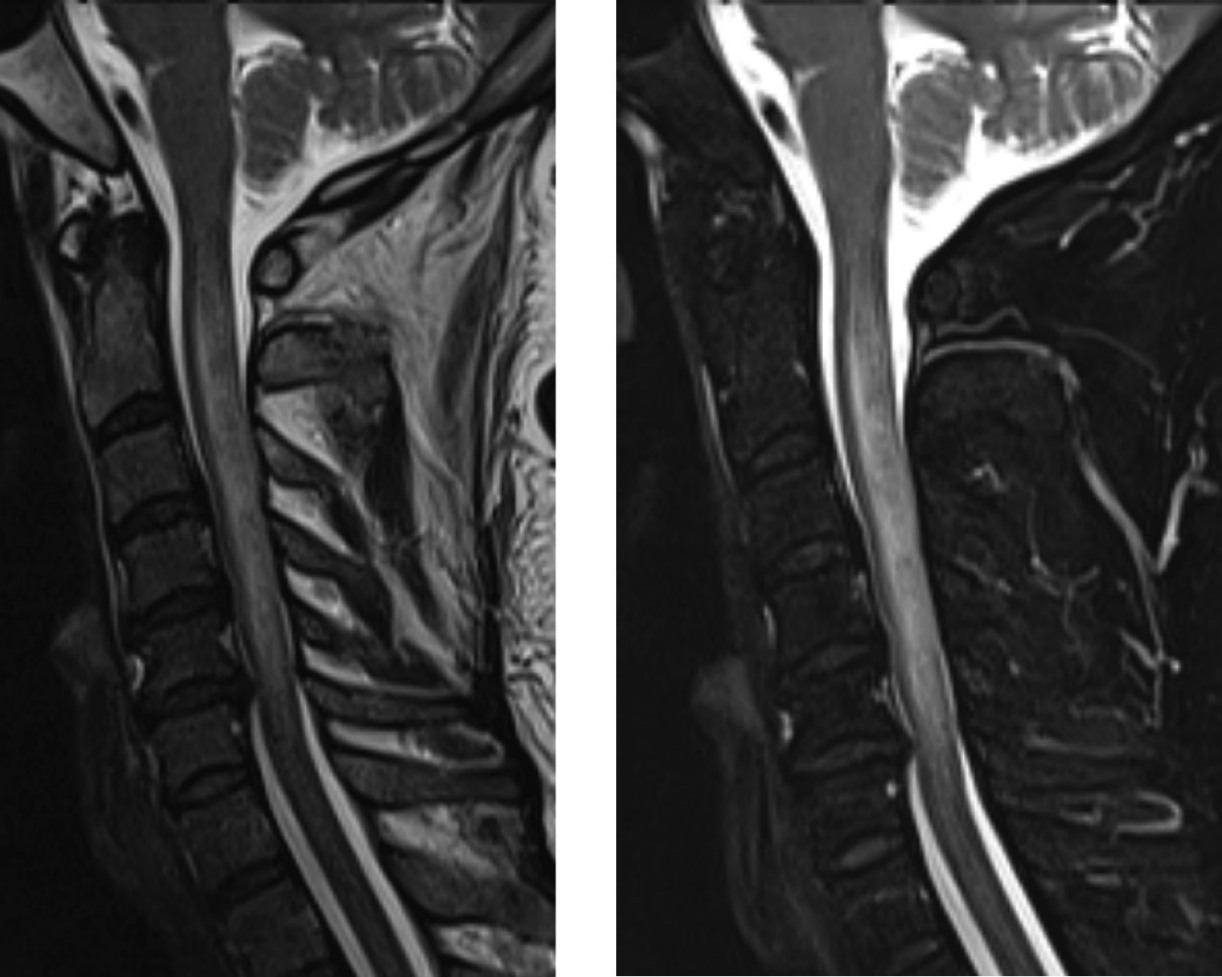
Sagittal TSE T2 (left) and T2 Dixon fat-saturated (right) MRI sequences of the cervical spinal show a swollen spinal cord associated with a T2-hyperintense signal extending from C2 to C5.
